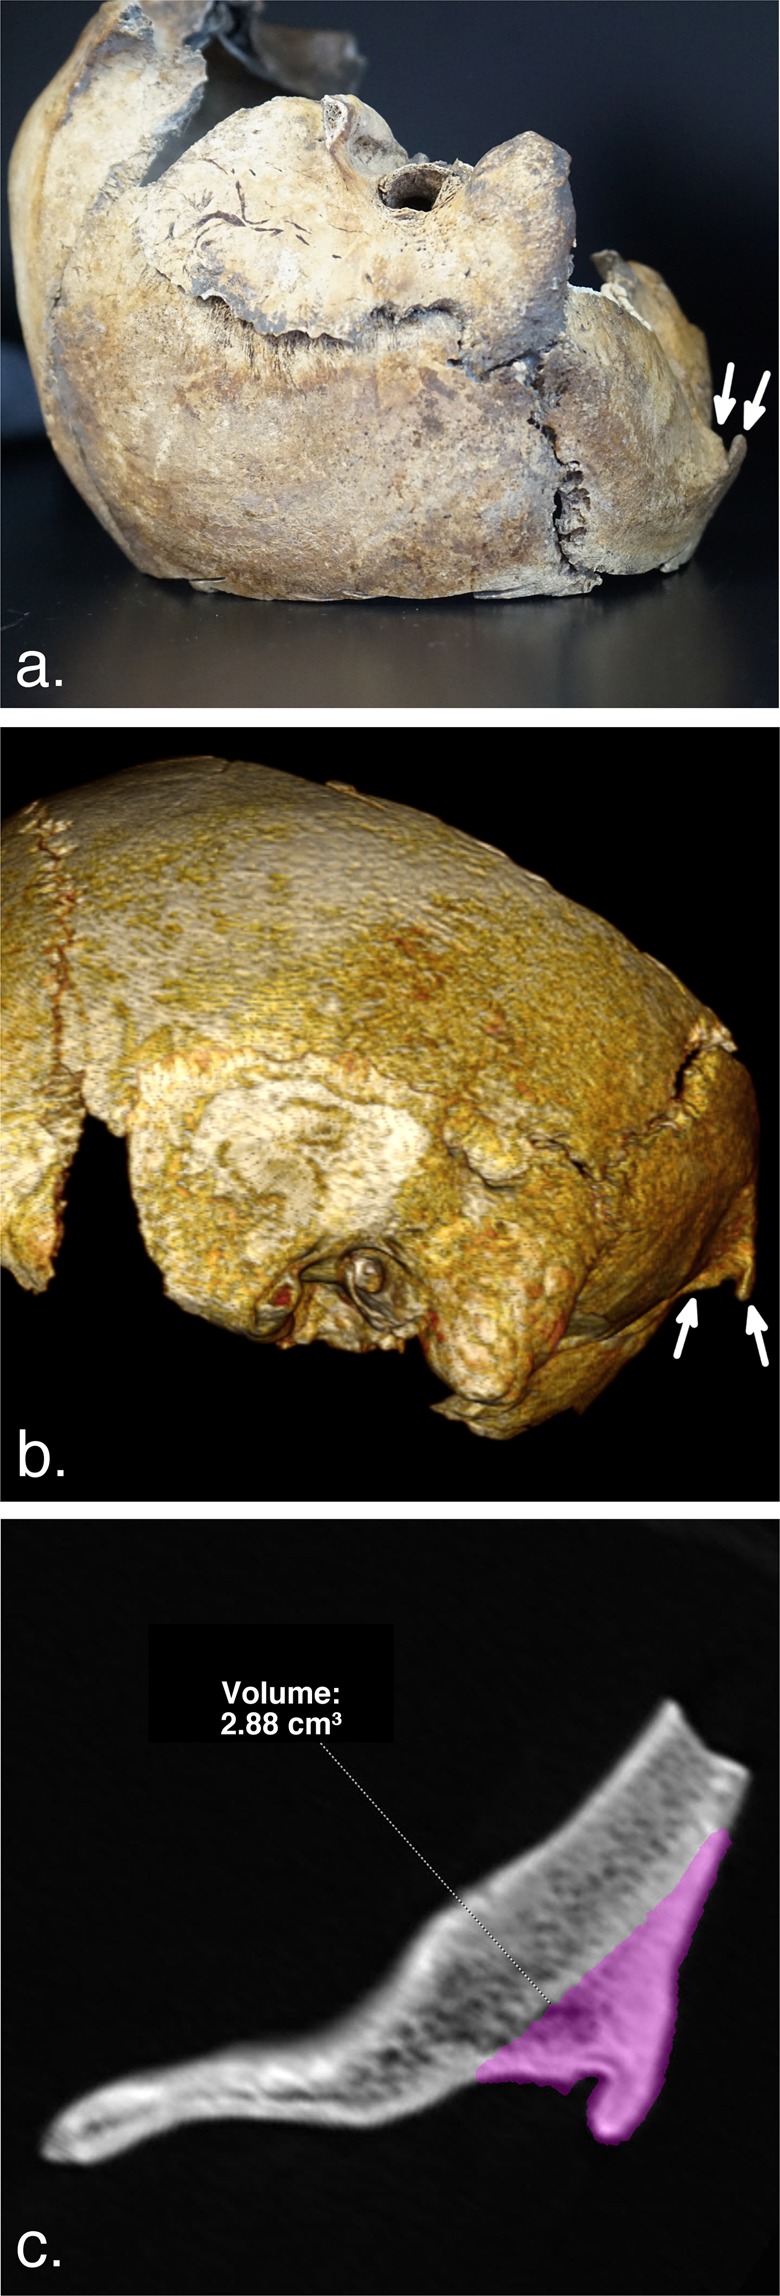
CT analysis of a young male’s skull from *circa* XVI^th^ century. Panel a. shows a lateral photograph of the skull, with visible enlarged type 3 EOP (arrows). Panel b. shows a lateral VR view of the skull with corresponding EOP (arrows). Panel c. shows EOP measurement (2.88 cm^3^), which corresponds to the 73^rd^ percentile of 2019 values for young men with EOP enlargement.
